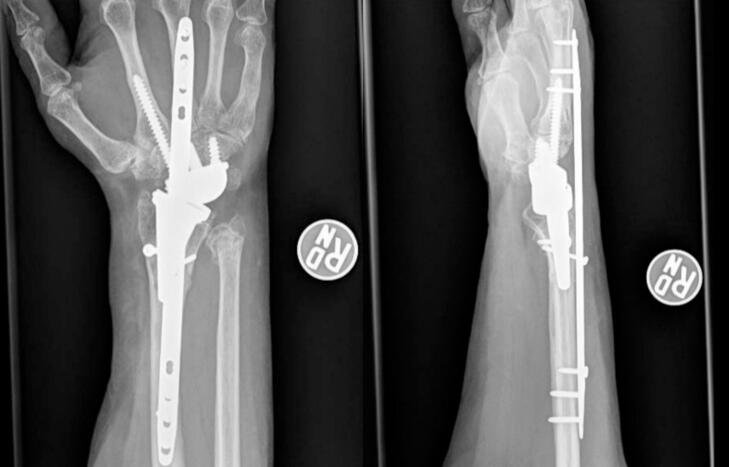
Right wrist radiograph 2.5 months post operatively demonstrating interval healing of radius and ulna fractures.
